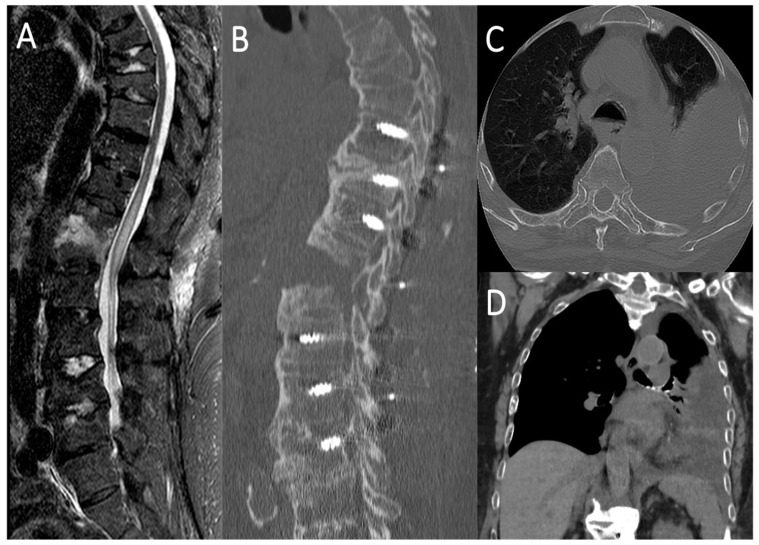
Spine MRI showing the T12 type B3 fracture with myelopathy of the conus medullaris (A); Post-operative CT-scan documenting the T9-L3 screw-rod fixation (B); Axial (C) and coronal (D) view of thoraco-abdominal CT-scan showing the massive left-sided pleural effusion.
